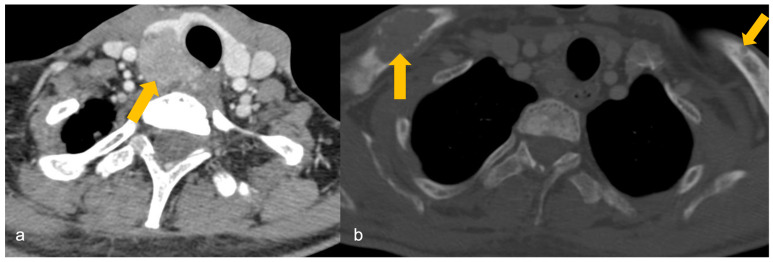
A 32-year-old male with a family history of parathyroid carcinoma presented with elevated serum calcium and parathyroid hormone. (a) Heterogeneously enhancing mass (arrow) arising posterior to the right lobe of thyroid gland on contrast-enhanced computed tomography (CECT), infiltrating the thyroid lobe and occupying the right trachea-oesophageal groove, findings suggestive of parathyroid carcinoma. (b) Osteolytic lesions in bilateral clavicles (arrows) on CECT, suggestive of biopsy-proven brown tumours.
